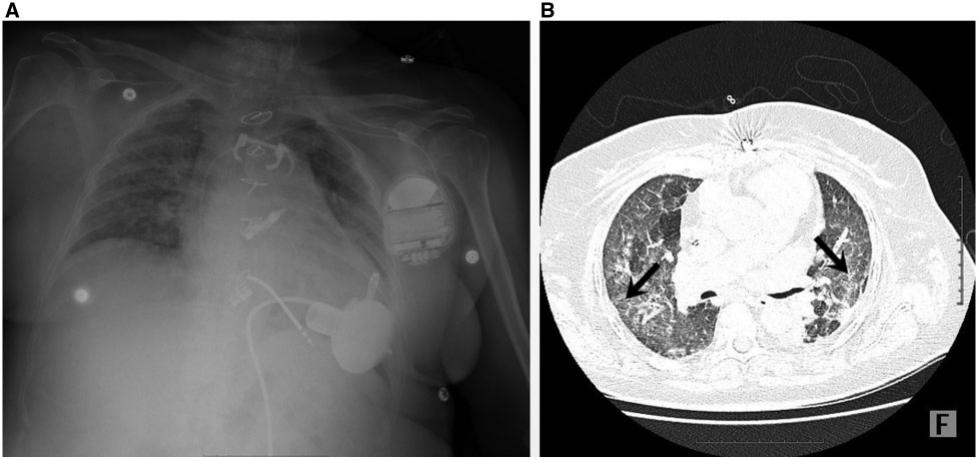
Radiological findings in the left ventricular assist device patient with positive COVID-19. (*A*) Chest X-ray demonstrated diffused bilateral opacities. (*B*) Computed tomography scan showed bilateral ground glass opacities and pulmonary consolidation.
